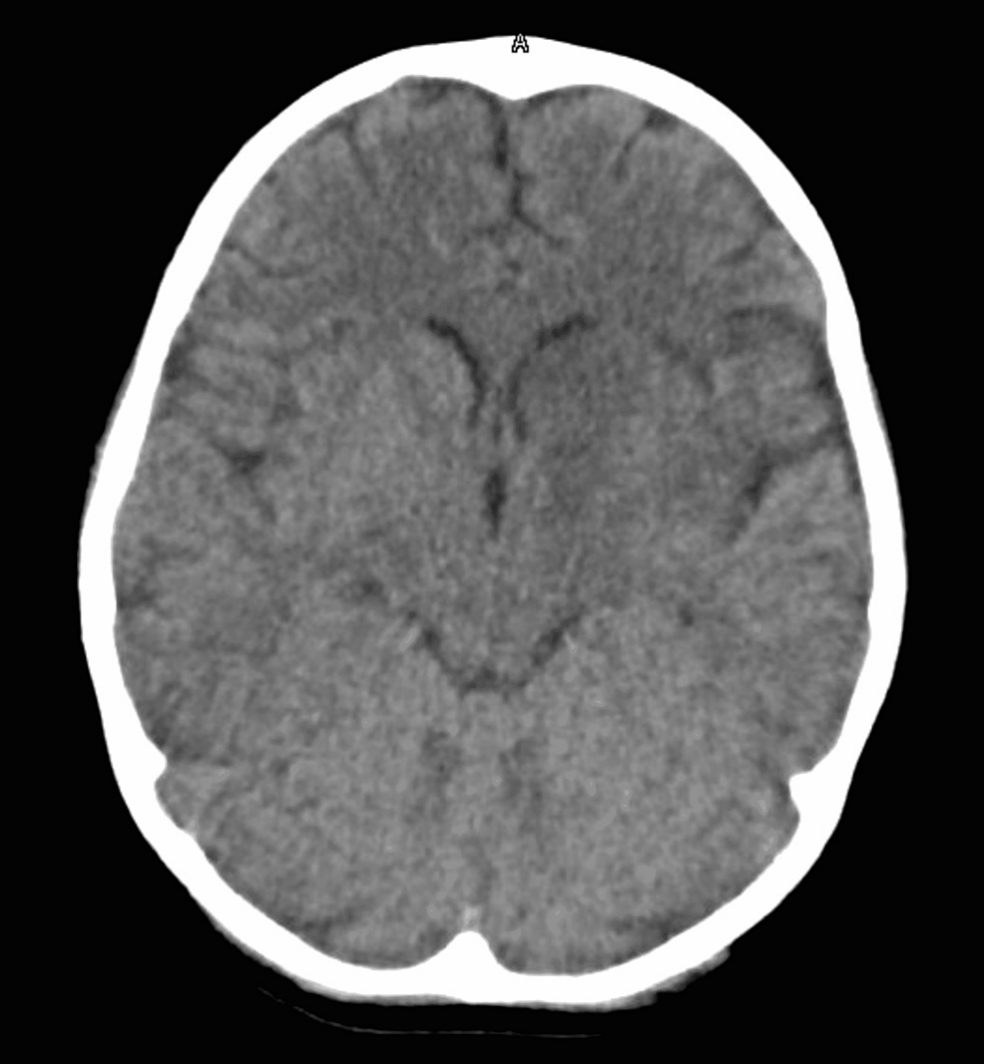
CT of the head obtained 72 hours after stroke No evidence of hemorrhagic conversion was noticed.
